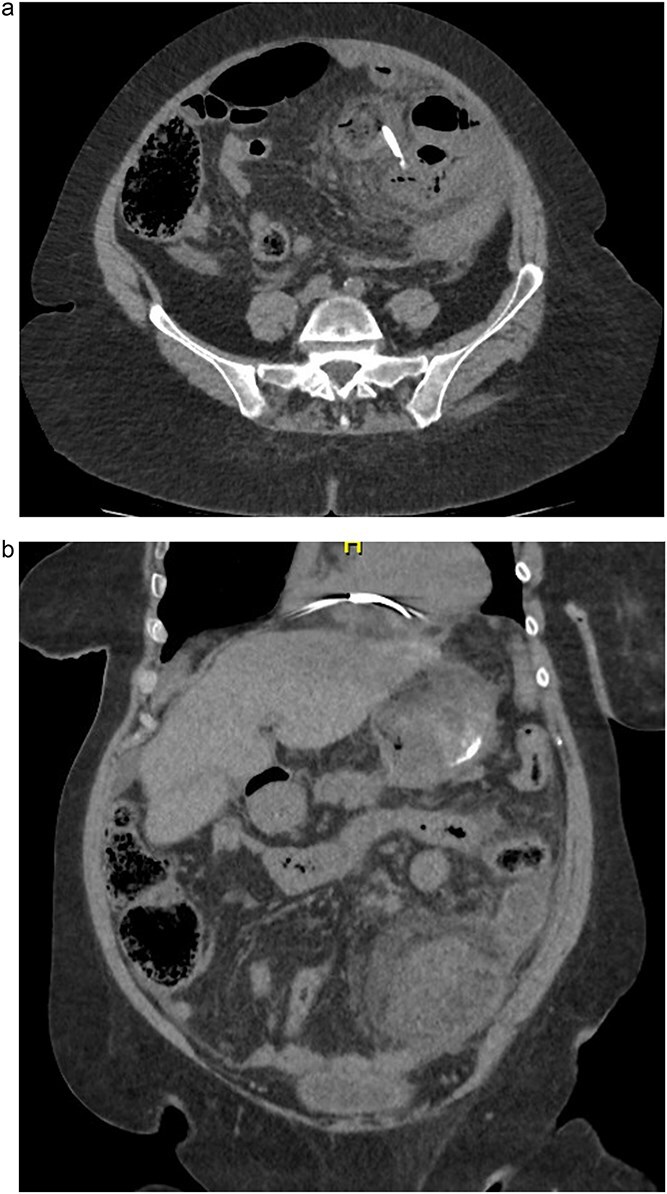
(a) CT scan of the abdomen and pelvis. Axial view showing a linear hyperdense foreign body within the small bowel loop, consistent with the migrated biliary stent. (b) CT scan of the abdomen and pelvis. Coronal view revealing an 8 cm fluid collection with rim enhancement adjacent to the jejunum, indicative of an intra-abdominal abscess secondary to perforation.
